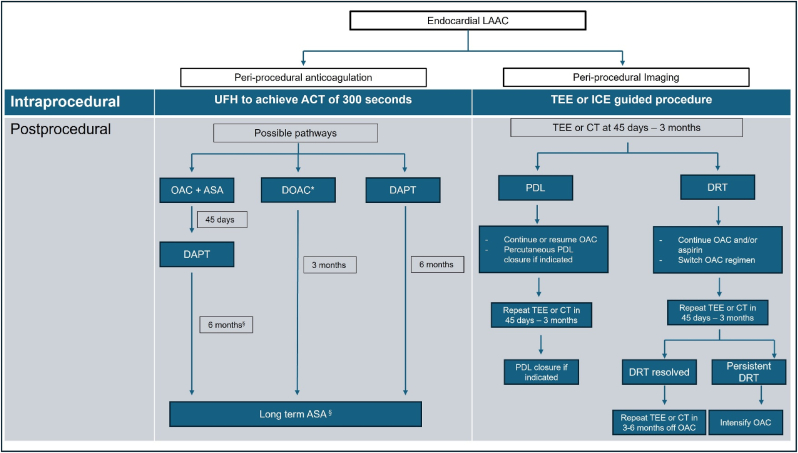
Antithrombotic Therapy and Imaging Surveillance After Left Atrial Appendage Closure ACT - Activated Clotting Time,ASA - Aspirin (Acetylsalicylic Acid), CT - Computed Tomography, DAPT - Dual Antiplatelet Therapy, DOAC - Direct Oral Anticoagulant, DRT - Device-Related Thrombus, ICE - Intracardiac Echocardiography, LAAC - Left Atrial Appendage Closure, OAC - Oral Anticoagulation, PDL - Peridevice Leak, TEE - Transesophageal Echocardiography, UFH - Unfractionated Heparin. ∗Can also consider half-dose DOAC plus aspirin (ASA). ^§^If no evidence of peridevice leak. ∗∗ OAC intensification – VKA (INR 2.5–3.5), low molecular weight heparin (LMWH), surgical excision if very large thrombus or OAC failure. If thrombus >15 mm, consider LMWH and repeat imaging in 2 weeks.
